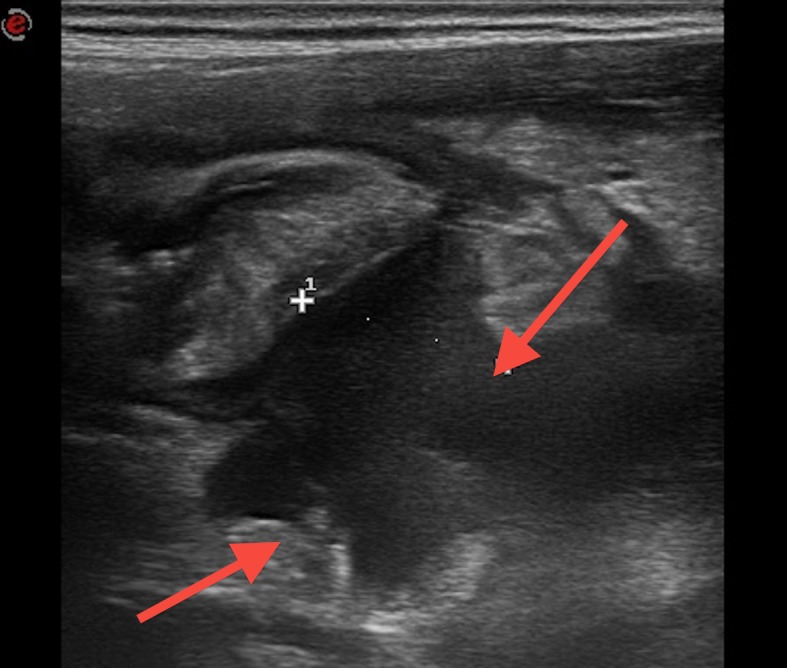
Figure 2:Abdominal ultrasound image showing a small amount of corpuscular pericecal free fluid extending to pouch of Douglas
